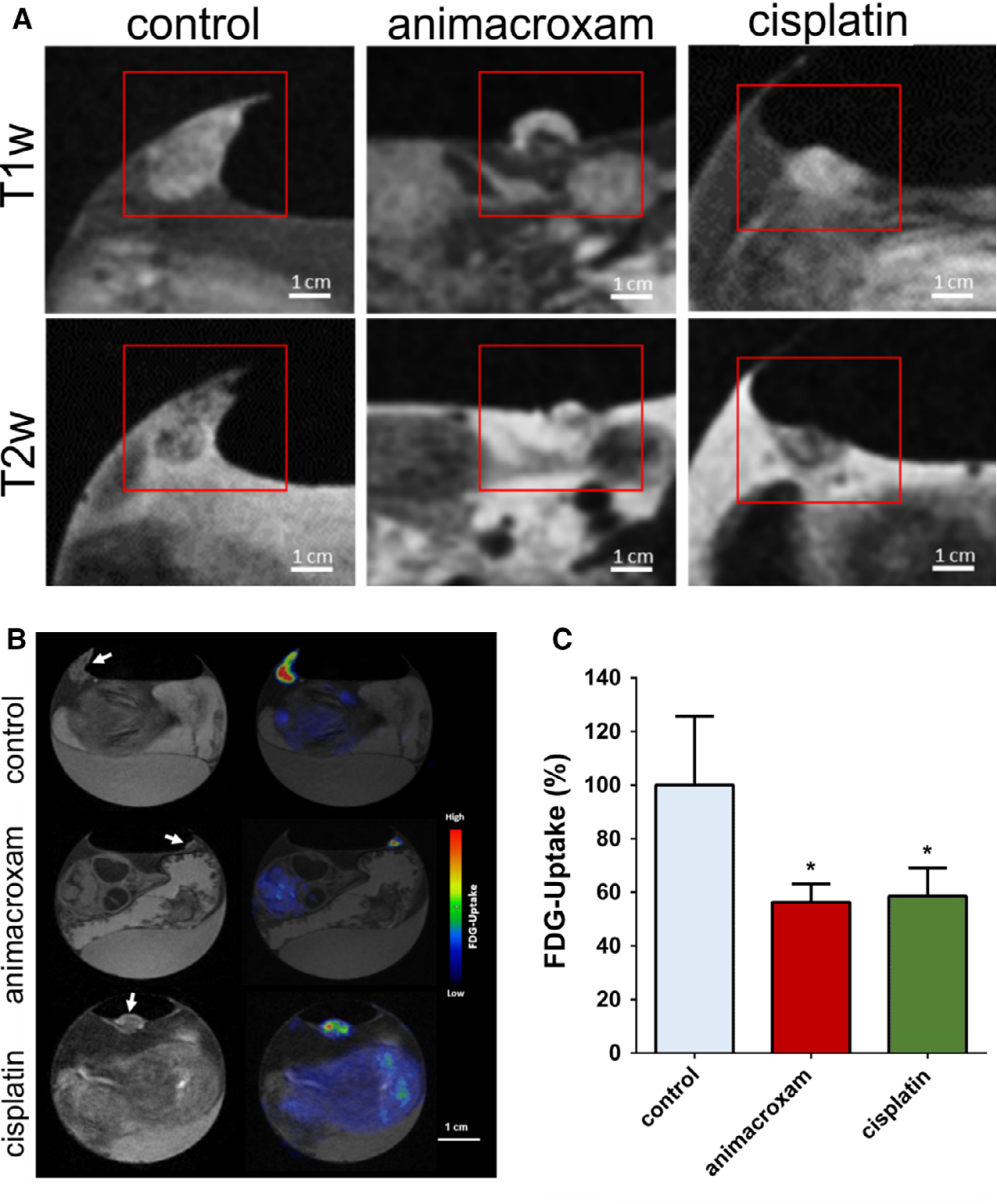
Animacroxam induces ‘cap formation’ and suppresses glucose uptake in inoculated TGCT plaques. (A) T1w and T2w images of control‐, animacroxam‐, and cisplatin‐treated tumor plaques. Animacroxam‐treated tumors show a distinct viable tumor core (dark in T1w‐/bright in T2w images) and a necrotic cap (bright in T1w‐/dark in T2w images). (B) T1w and corresponding PET‐MR images of 18F‐FDG uptake in tumor plaques treated with NaCl, animacroxam, or cisplatin. (C) Animacroxam (5.0 µm)‐ and cisplatin (2.5 µm)‐treated tumor plaques showed a reduced uptake of 18F‐FDG. Results are shown as means ± SEM of at least *n* = 3 independent experiments. Scale bar = 1 cm. P*‐values of ≤ 0.05, unpaired *t*‐test.
